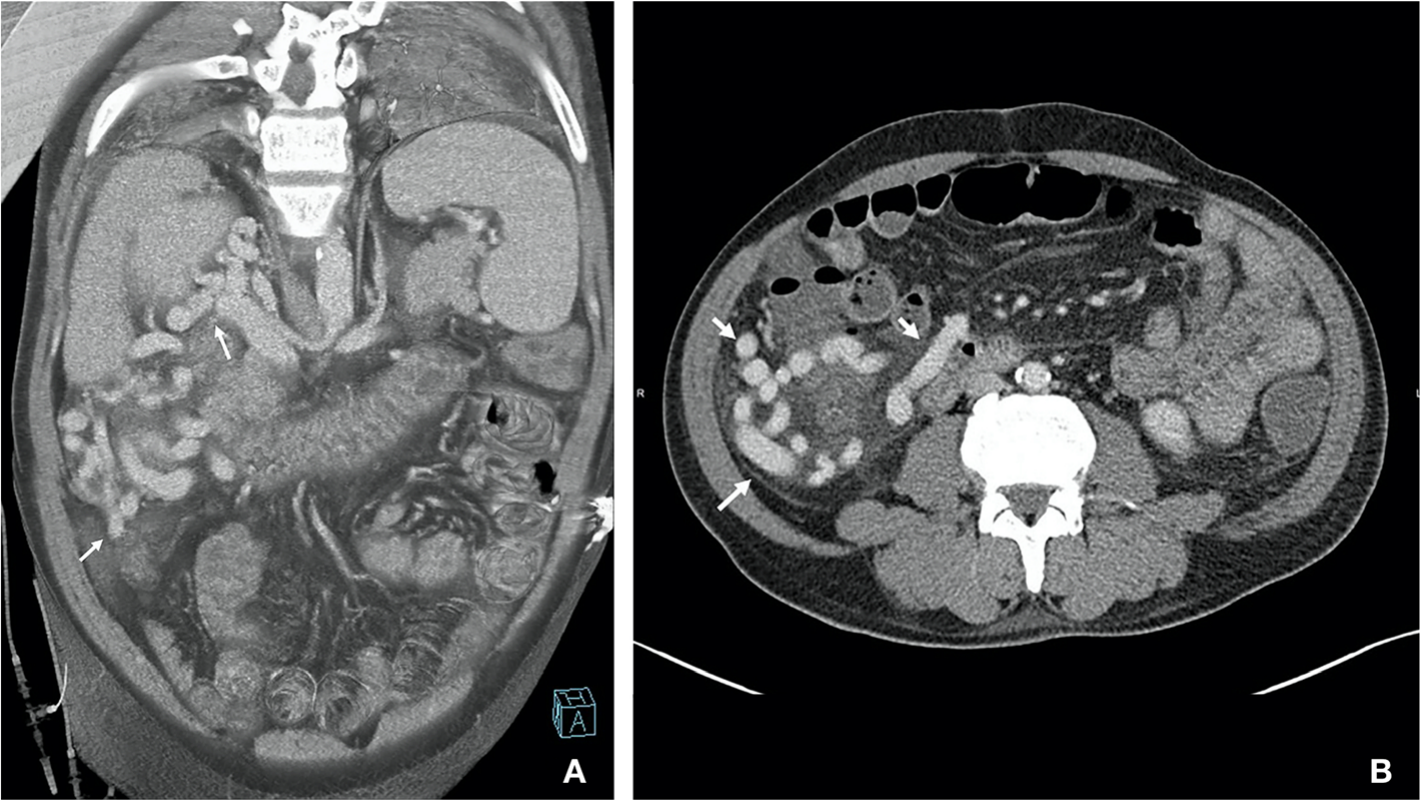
a Coronal thin MIP image from portal venous phase showing pericolic varices (2 white arrows) with dilated right renal vein. b Axial CT image in portal venous phase showing large peri-colic collaterals around the ascending colon (3 white arrows)
